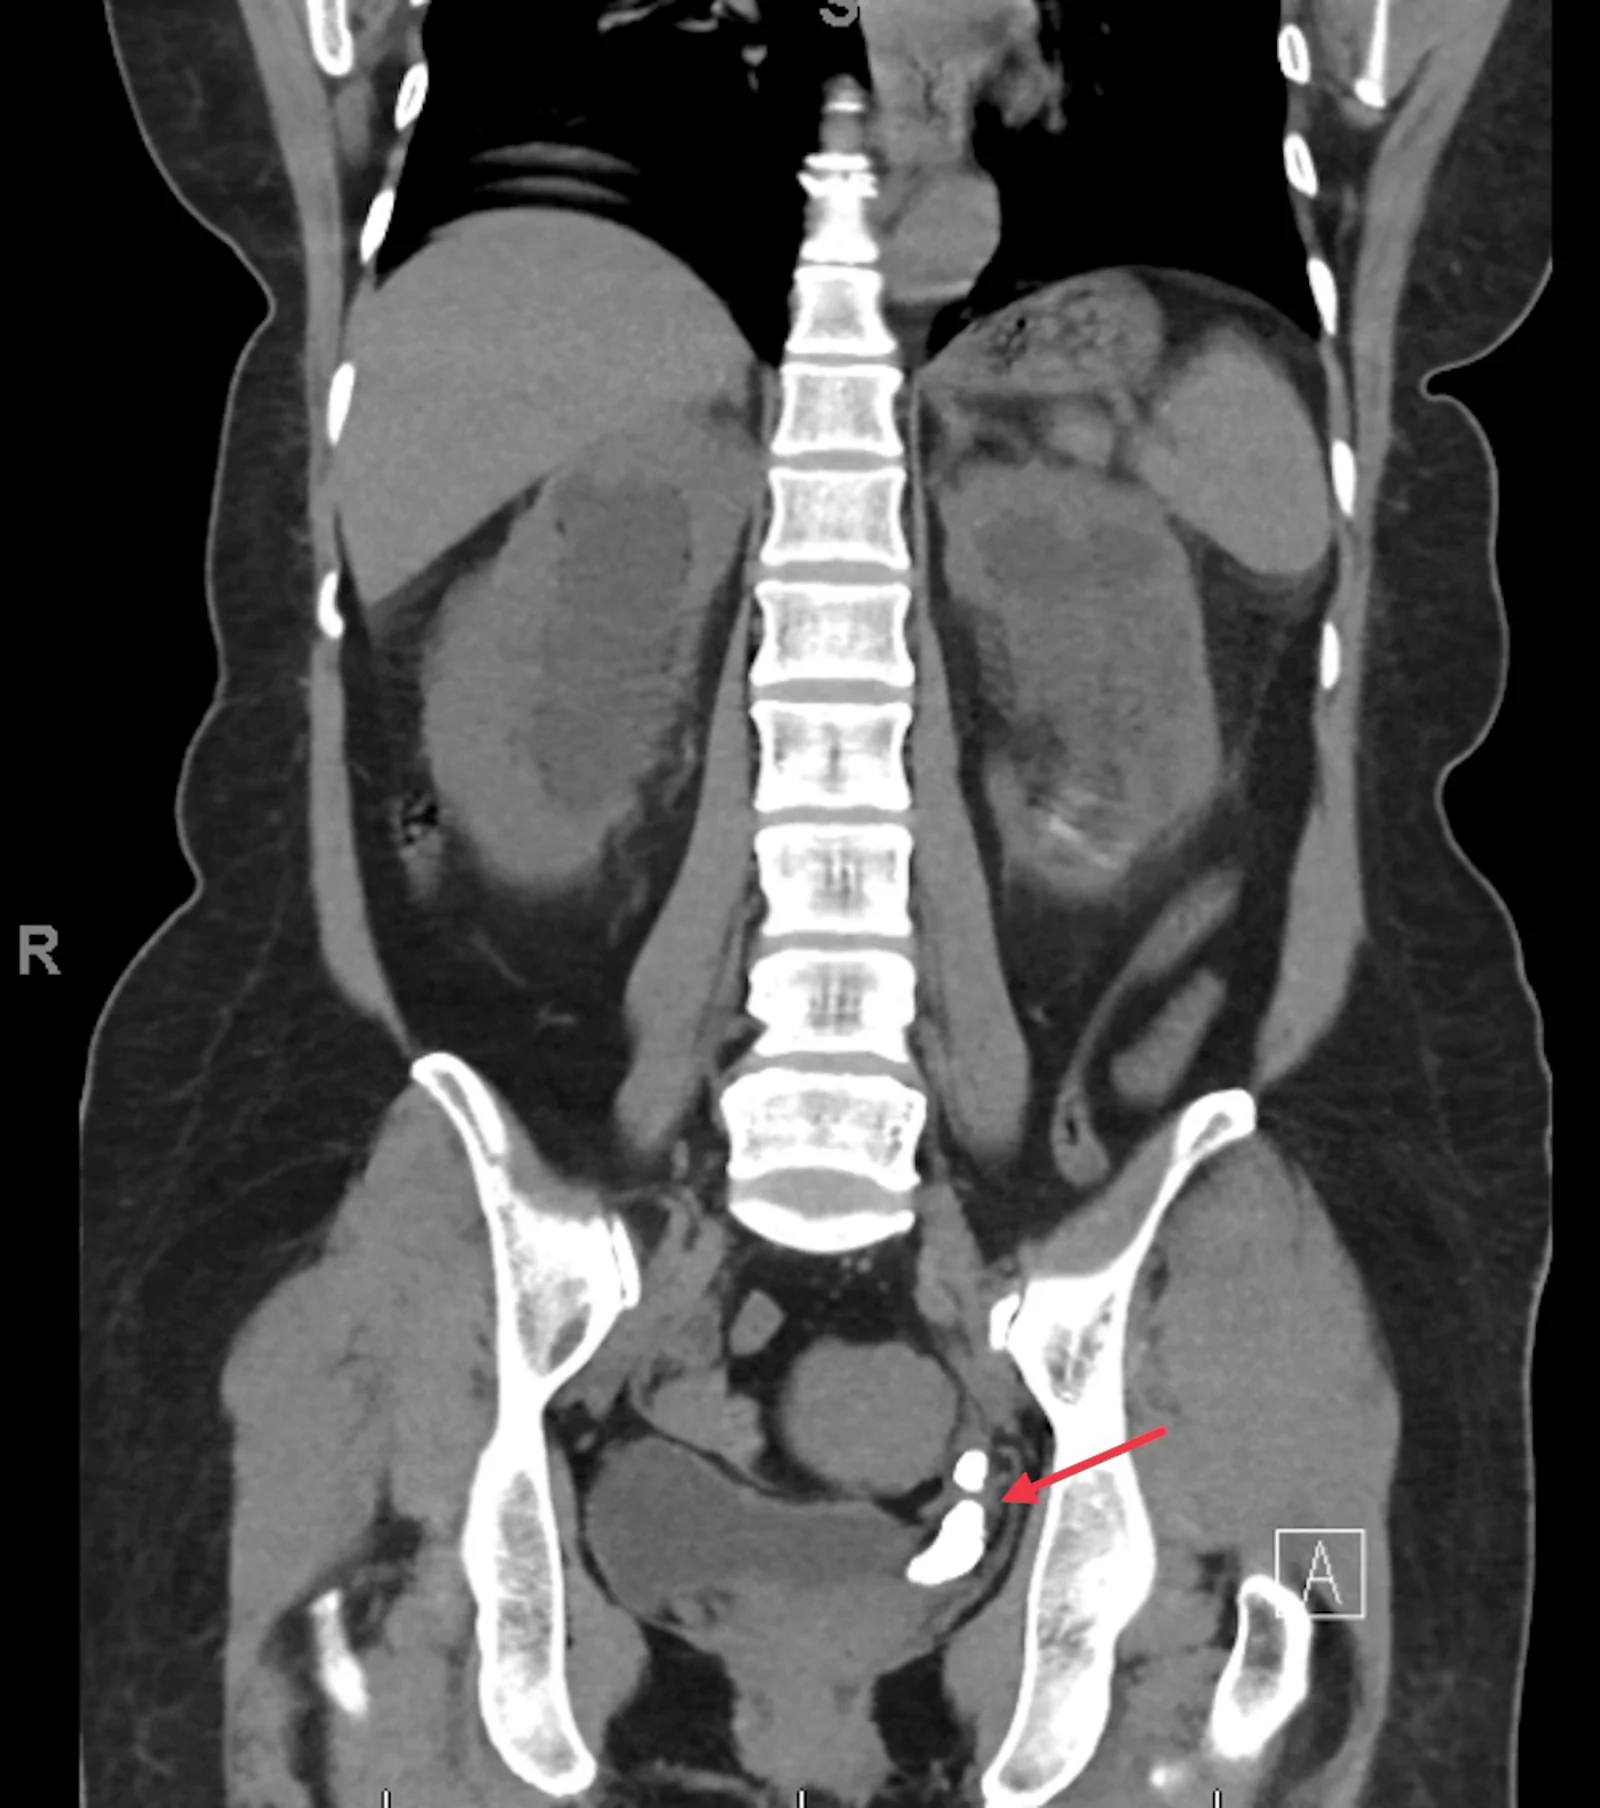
Coronal non-contrast CT image showing left distal ureteral stones. The red arrow points to the stone.
